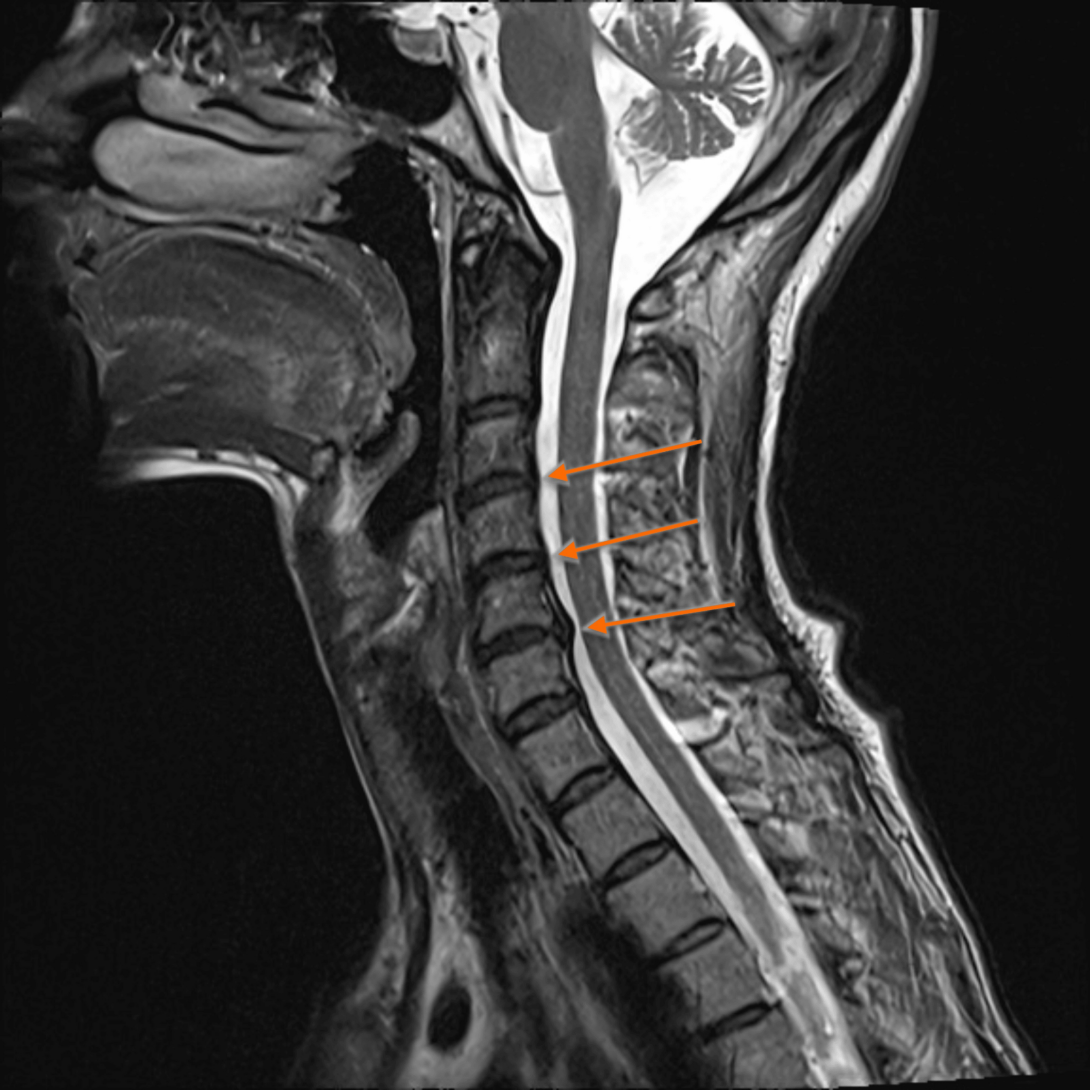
T2-weighted sagittal MRI of the cervical spine demonstrating moderate degenerative disc changes at C3-C4 and C4-C5, and disc bulge at C5-C6 (orange arrows). MRI: magnetic resonance imaging.
